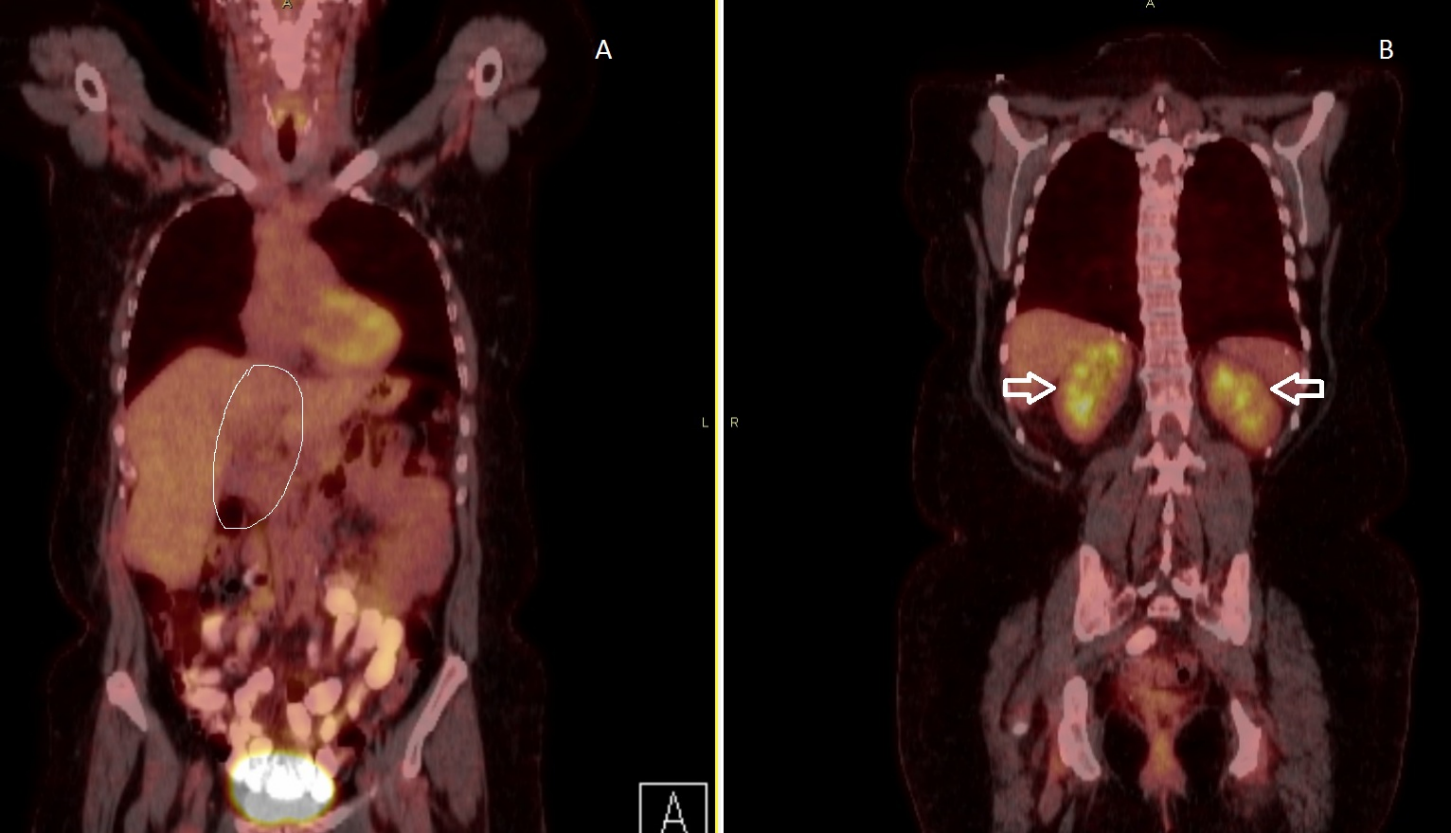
A PET/CT in October 2016 revealed no focal abnormality in the resection bed (outlined in Figure 4A), no gross evidence of metastatic disease, no significant retroperitoneal or intra-abdominal lymphadenopathy. Normal uptake was noted in bilateral kidneys (short arrows in Figure 4B). PET/CT - Positive emission tomography/computed tomography.
